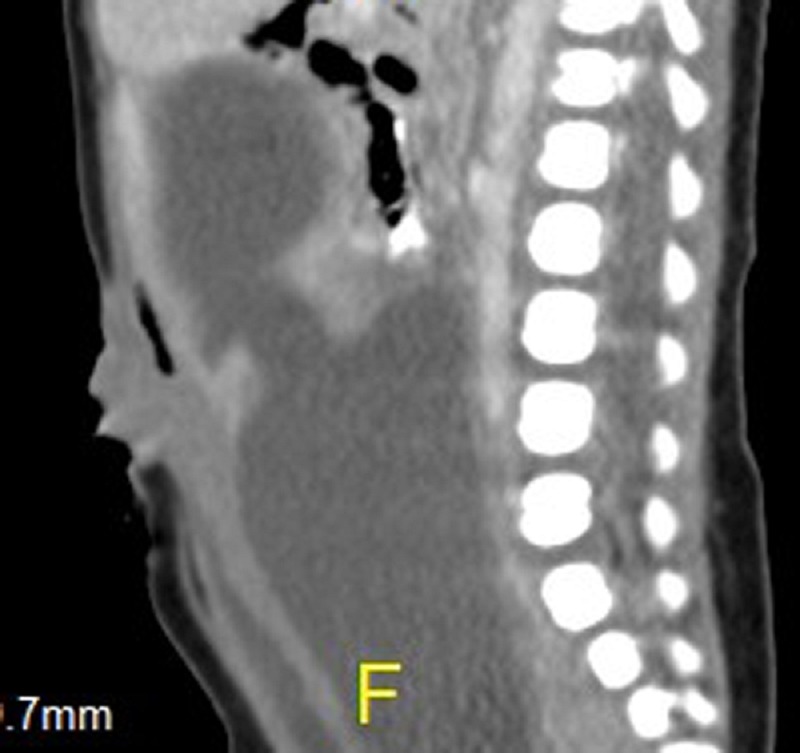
Figure 1: CT-Scan showing hydrometrocolpos. Note the dilated vagina and uterus.
